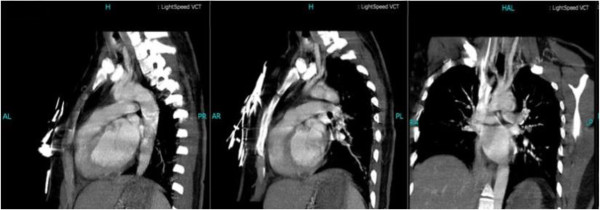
Thoracic and abdominal computed tomographic angiography (CTA) was negative.
